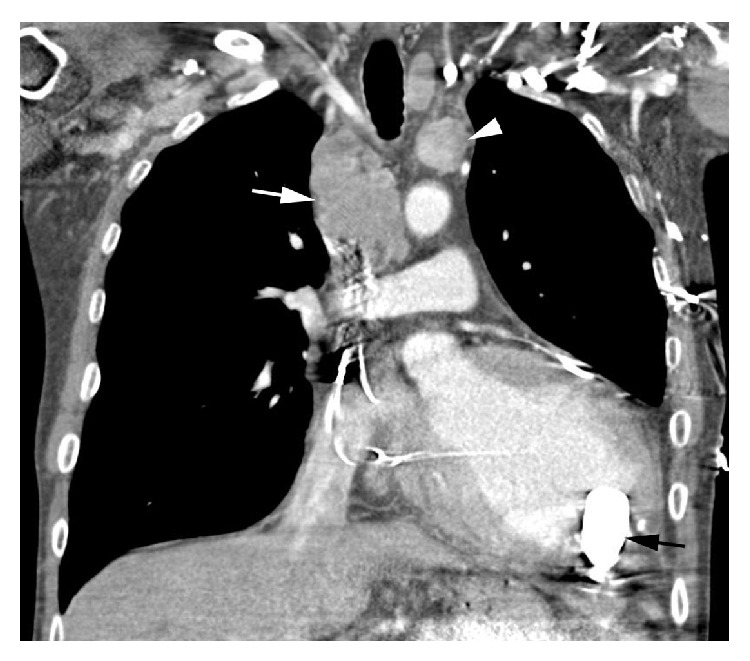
Coronal IV contrast-enhanced CT image shows right (white arrow) and left (white arrowhead) paratracheal lymphadenopathy. LVAD inflow cannula within the left ventricle (black arrow).
